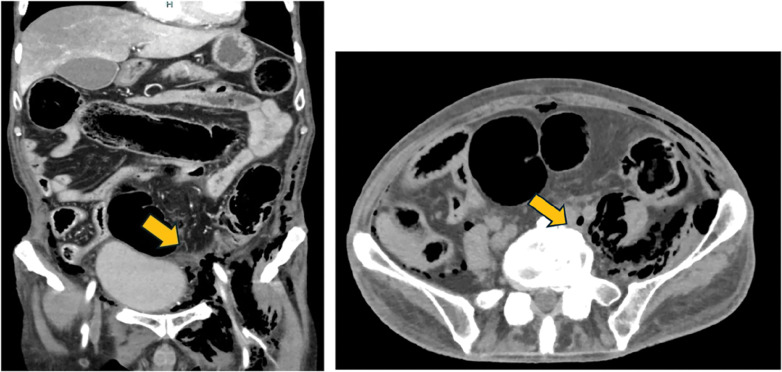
CT scan from the referring hospital. The yellow arrows indicate an abscess in the abdominal cavity.
